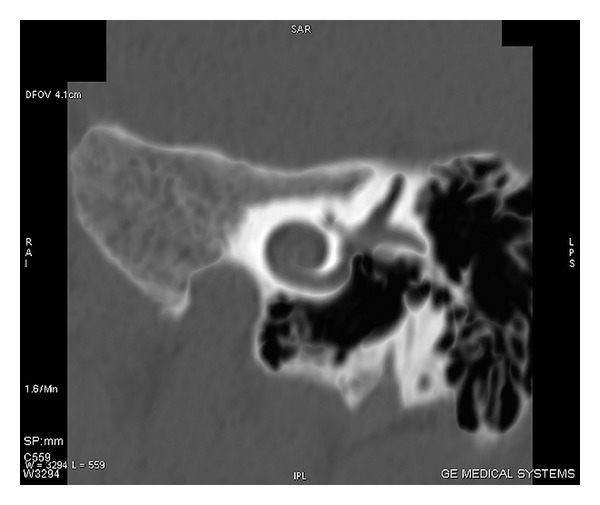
HRCT scan of temporal bone with coronal section showing cochlear anomaly in which cochlea shows 1 and 1/2 turns.
